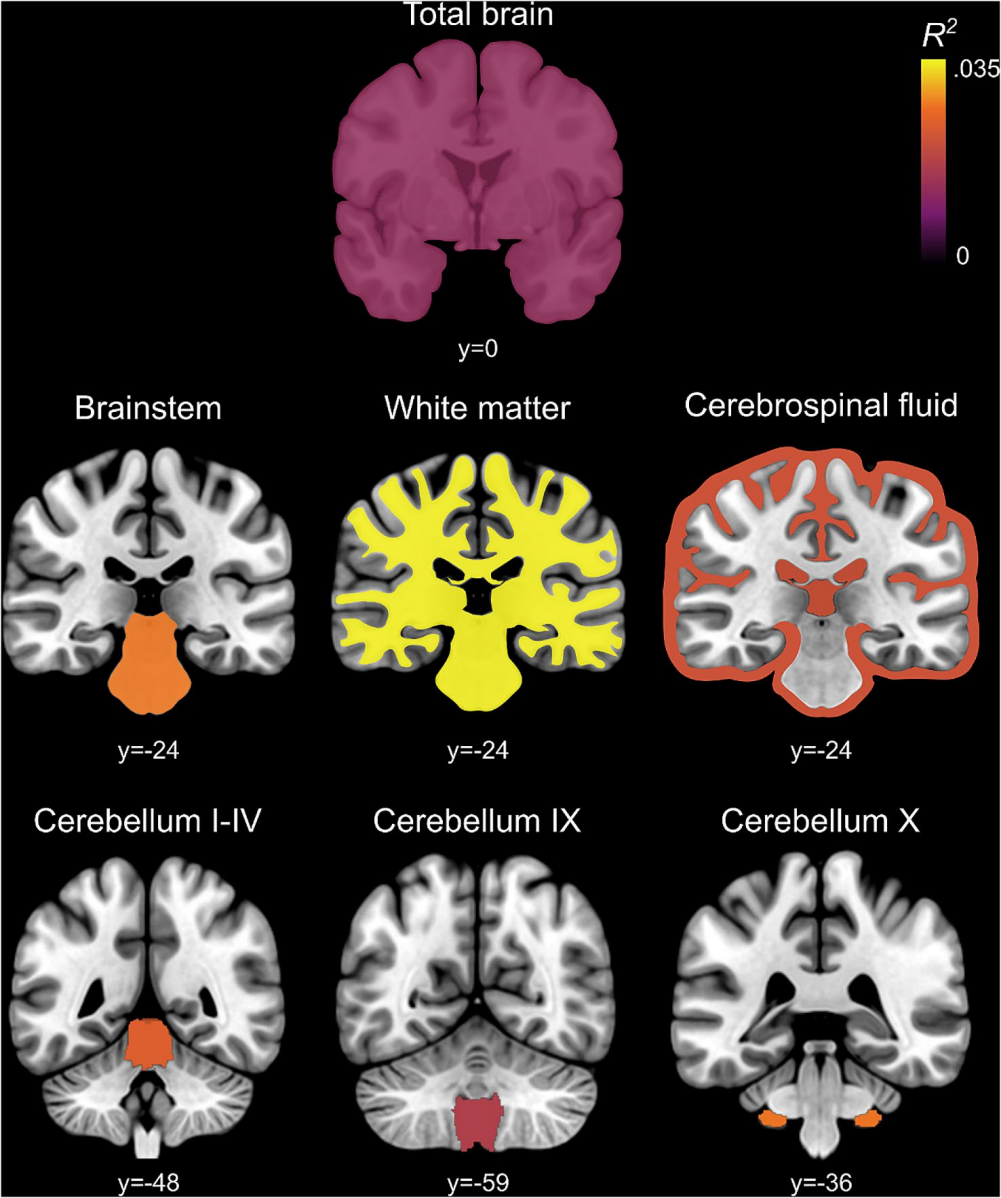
Anatomical localization of polygenic risk effects for ASD on the primary MRI phenotypes, *Total Volumes*. Coronal brain slices depicting the significant association between the total volumes and ASD PRS. The color bar indicates the highest variance (*R*^*2*^) explained by the ASD PRS for the primary MRI phenotypes with significant associations
